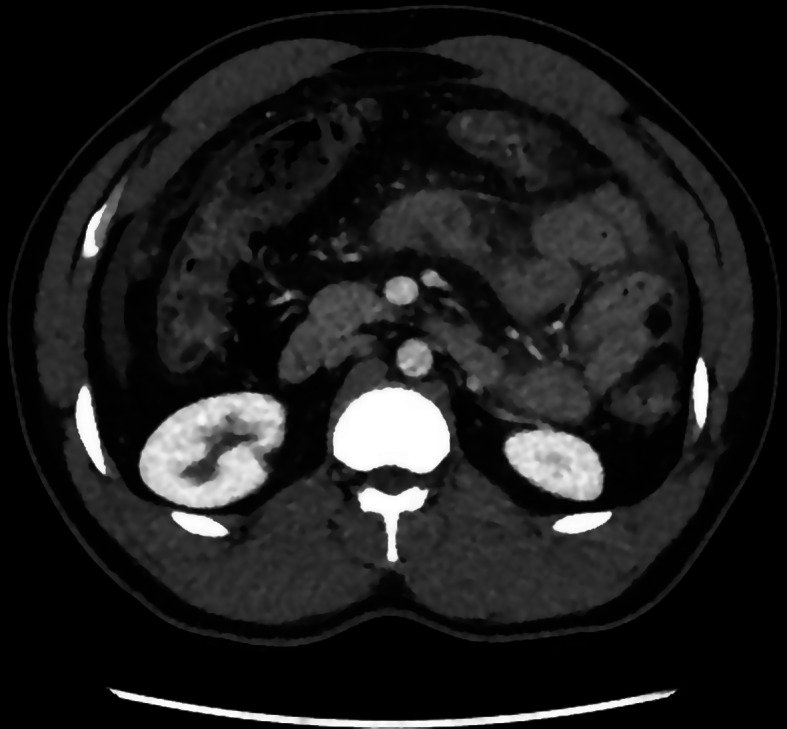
First CT scan with free fluid, reactive mesenteric lymph nodes, peritoneal thickening, mesenteric fat infiltration, wall thickening of the colon and segmental wall thickening of the jejunum
